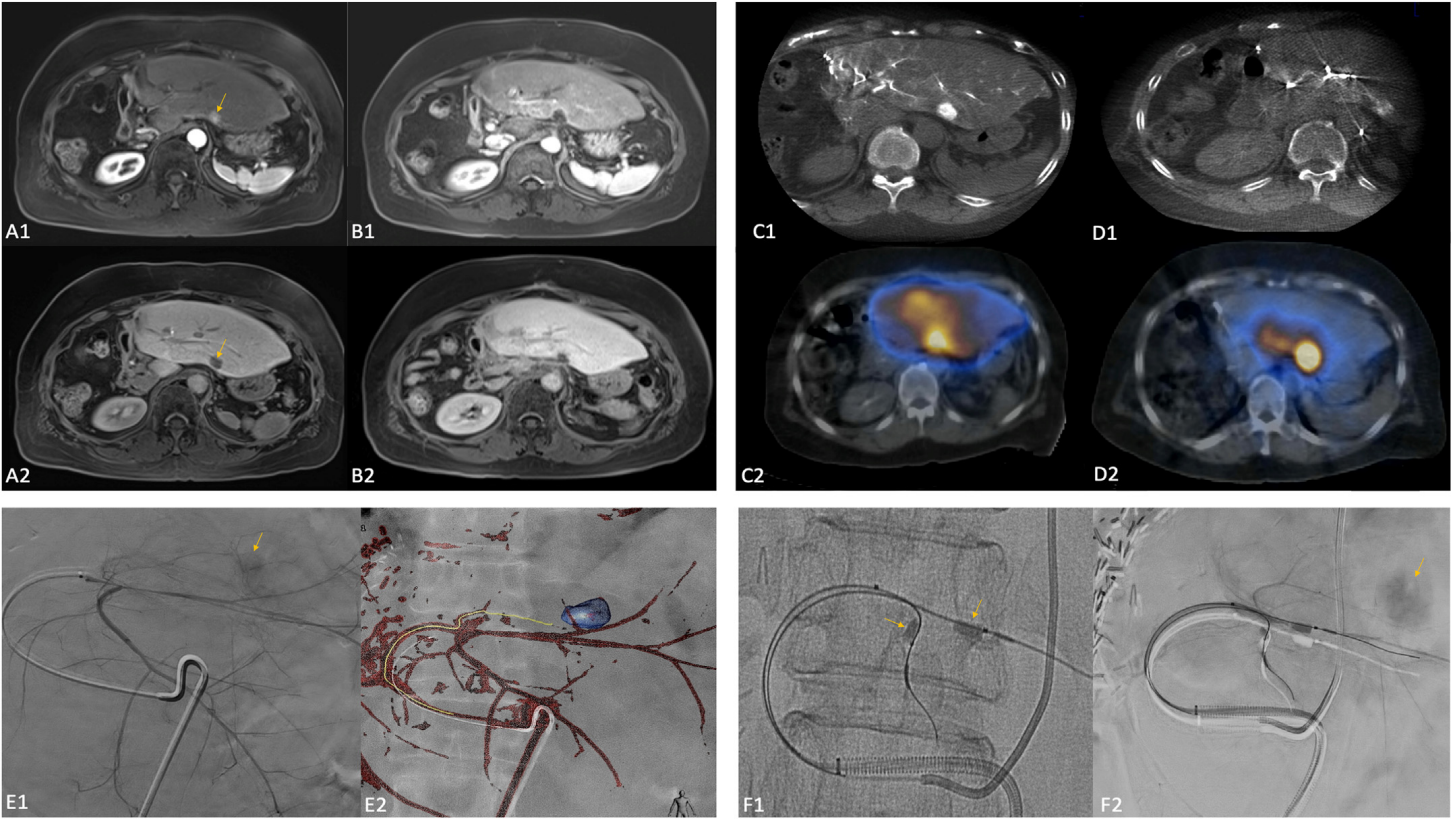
Case 1.68 year old female patient with HCC status post right hepatectomy with tumor recurrence in the left hepatic lobe as demonstrated on arterial phase and hepatobiliary phase MRI (A1 and A2, arrows). Left hepatic angiography (E1 and E2) as well as CBCT and SPECT-CT (C1 and C2) from the MAA mapping procedure demonstrated enhancement/uptake of the target segment (arrow) as well as the surrounding parenchyma. CBCT and SPECT-CT after balloon occlusion of the distal segment 2 and 3 arterial branches (F1 and F2, arrows) demonstrated augmented flow to the tumor (arrow) and decreased flow to the surrounding parenchyma. Follow-up arterial phase and hepatobiliary phase MRI one year post-treatment (B1 and B2) demonstrated complete response and minimal radiation effect to the surrounding parenchyma.
